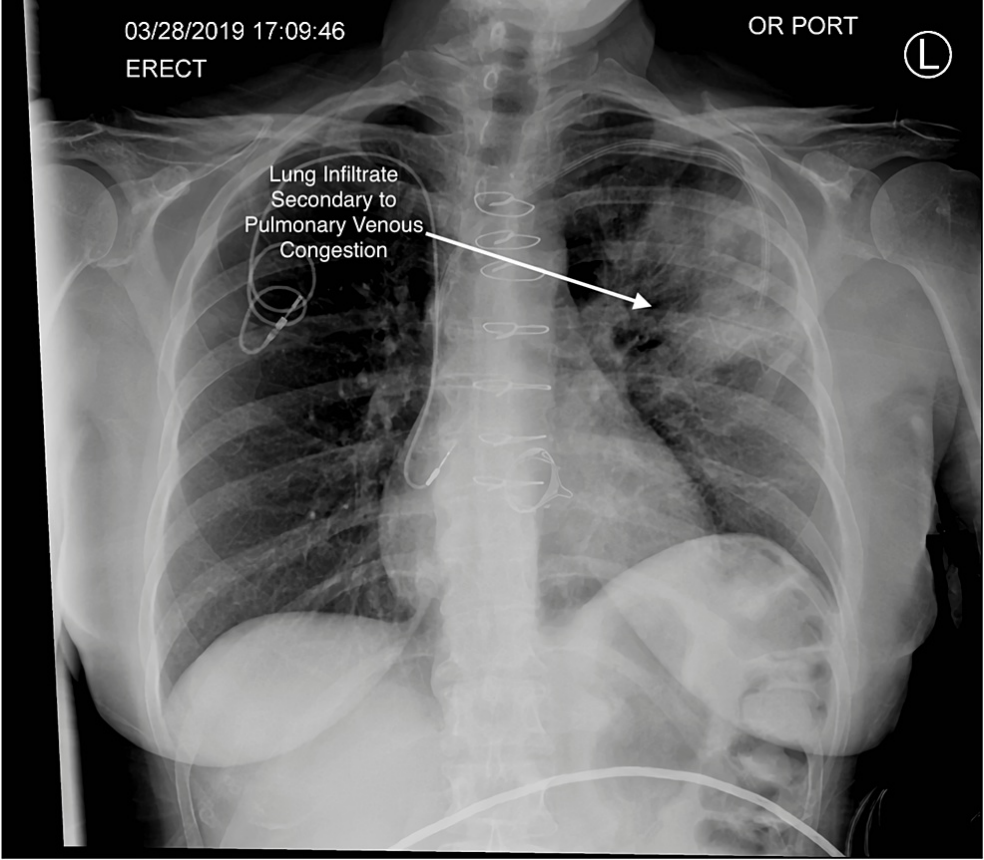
Chest X-ray demonstrating new parenchymal opacities in the left upper lobe secondary to pulmonary venous obstruction.
